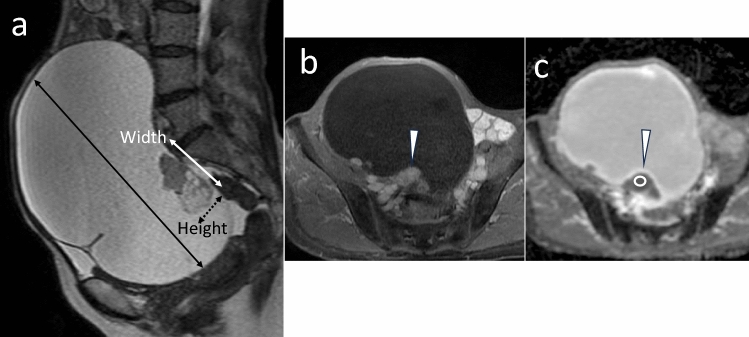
Clear cell carcinoma with FIGOIA in a 66-year-old woman. a Sagittal single-shot fast-spin echo T2-weighted image showing the largest dimension with the height and width of the maximal solid component. The size of the maximal solid component was measured: height, 25 mm (a, dotted blackline) and width, 49 mm (a, white line with bilateral arrowheads). The height–width ratio was 0.510. The maximal lesion size (a, black line with arrowheads) was 182 mm. b Axial contrast-enhanced T1-weighted image showed solid area (arrowhead) of the maximal solid component. The ADC value (1.54 × 10^–3^ mm^2^/sec) was measured placing a round two-dimensional (50 mm^2^) region of interest (ROI) manually in the solid area of the maximal solid component. Cystic, hemorrhagic and necrotic areas were avoided
